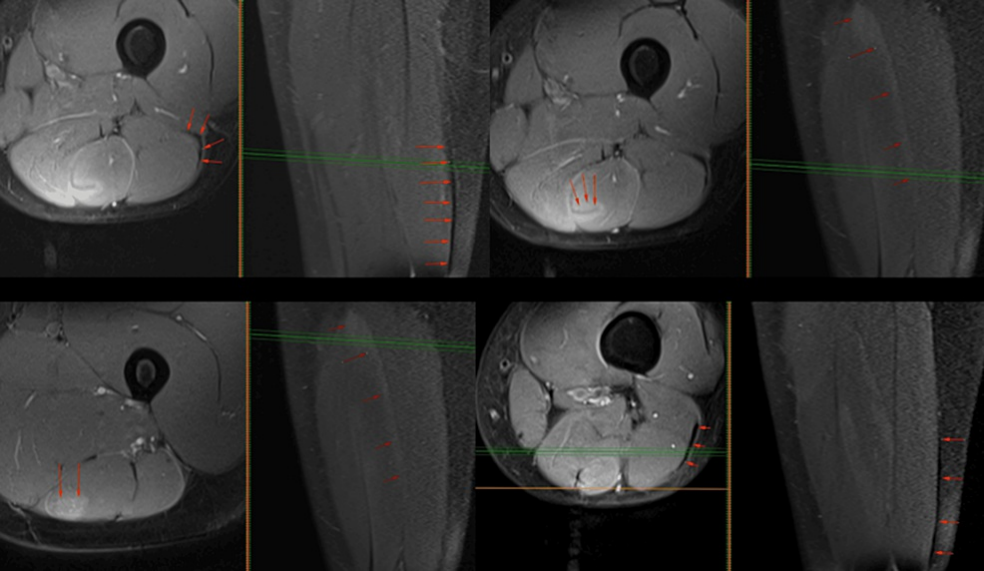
T2-weighted MRI imaging of the left thigh: transverse and coronal planes - para-aponeurotic edema within the semitendinosus muscle, particularly concentrated in the intramuscular raphe and aponeurosis of the distal musculotendinous T-junction (DMTJ) (red arrows).
